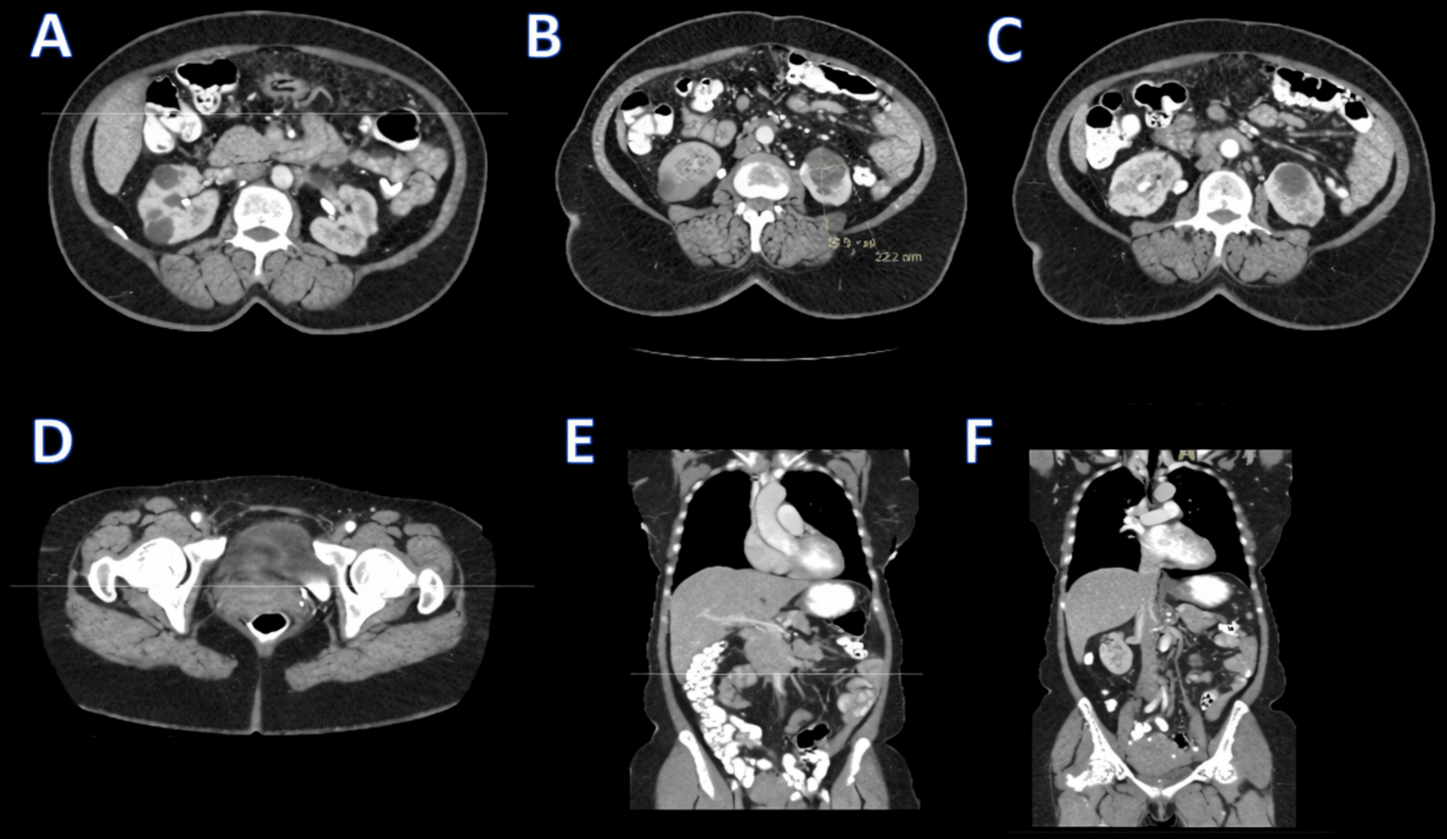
Post-treatment contrast-enhanced CT demonstrating complete radiologic resolution of prior peritoneal disease (A–C) Axial contrast-enhanced CT images of the abdomen demonstrating normal appearance of the liver, bowel, mesentery, and omentum without evidence of ascites, peritoneal thickening, or omental caking. (D) Axial CT image of the pelvis demonstrating normal uterus and adnexa without pelvic masses or free fluid. (E–F) Coronal CT reconstructions of the abdomen and pelvis confirming the absence of ascites, lymphadenopathy, peritoneal disease, or solid-organ masses.
